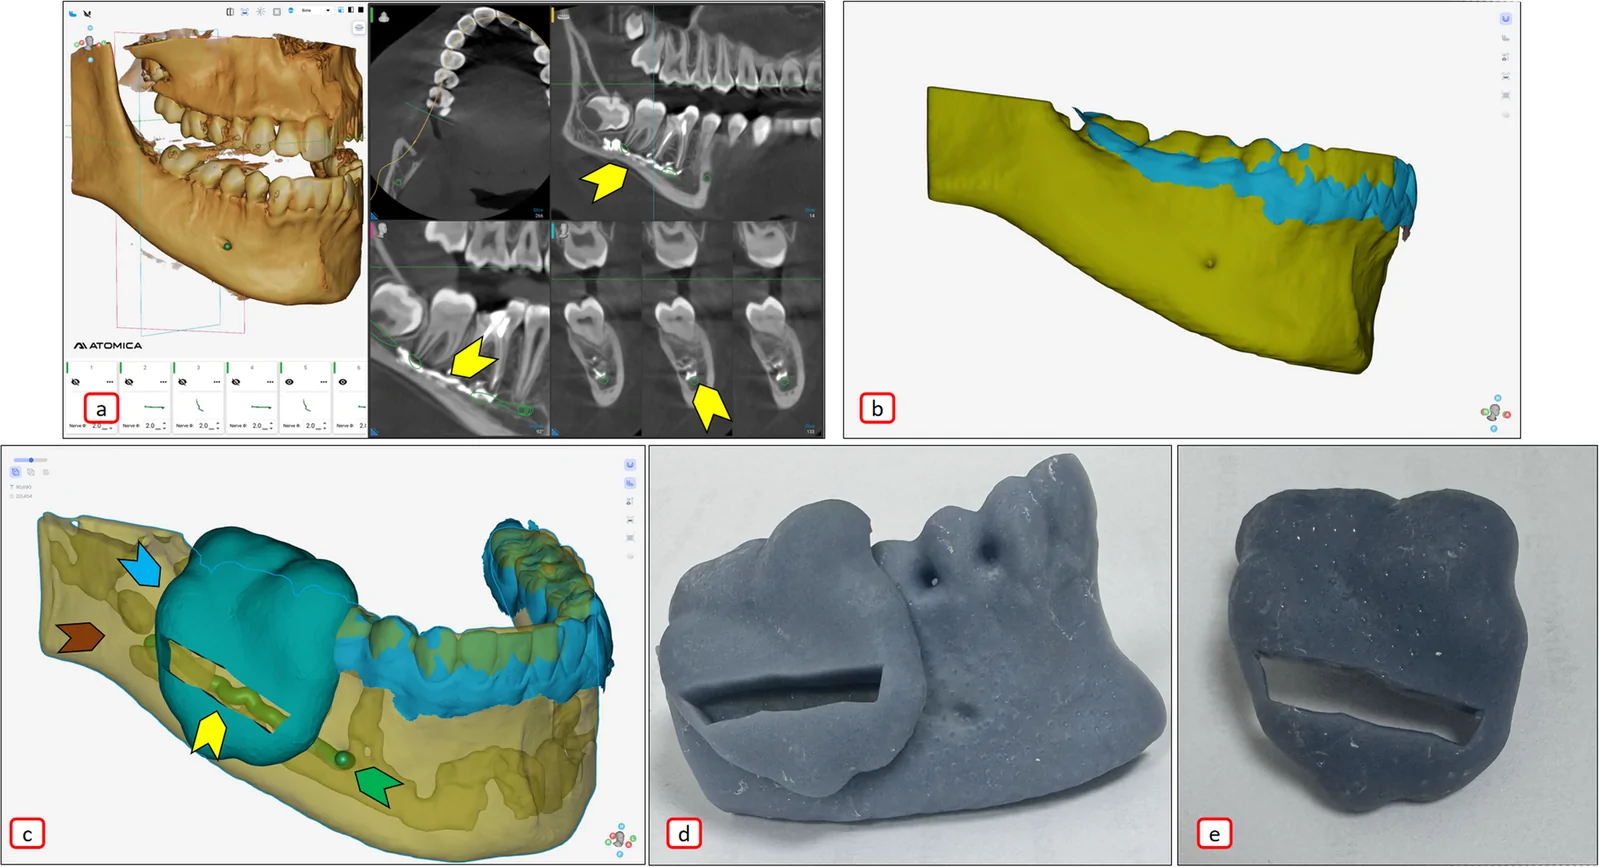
Diagnostic Imaging and Preoperative Virtual Planning. a Preoperative cone-beam computed tomography (CBCT) panoramic reconstruction showing radiopaque bioceramic sealer (arrow) extruded into and occluding the course of the right inferior alveolar canal. b Superimposition of the intraoral digital scan onto the CBCT data-set for integrated three-dimensional treatment planning. c Virtual design of the patient-specific, tooth- and bone-supported surgical guide in the planning software (highlighted with blue arrow), outlining the planned osteotomy window (highlighted with yellow arrow). d Three-dimensional bone model demonstrating the planned relationship of the surgical guide to the lateral cortex of the mandible and the underlying inferior alveolar canal. e The finalized surgical guide fabricated via stereolithography (SLA) 3D printing in a medical-grade resin
